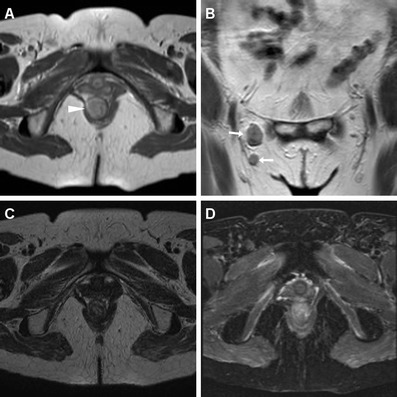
A 46-year-old female patient with biopsy-proven SCAC. MRI staging including axial (a) and coronal (b) T2-weighted images detect a solid, 3-cm eccentric anal mass (*arrowhead*) consistent with T2 tumour, associated with right inguinal adenopathies with analogous signal features. After chemo-radiotherapy, MRI follow-up (c, d) shows complete disappearance of both anal neoplastic solid tissue and lymphadenopathies
